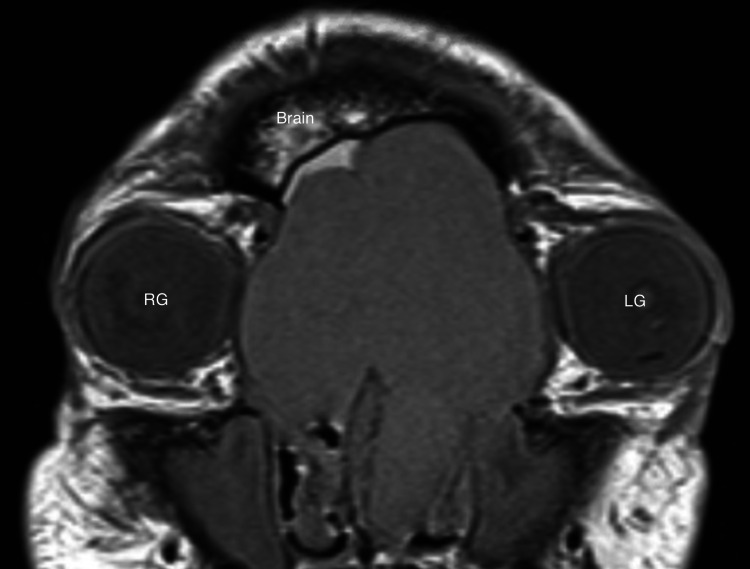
MRI on presentation, coronal view, T1 MRI with gadolinium demonstrating mass effect on skull base and globes. RG: right globe: LG: left globe.
